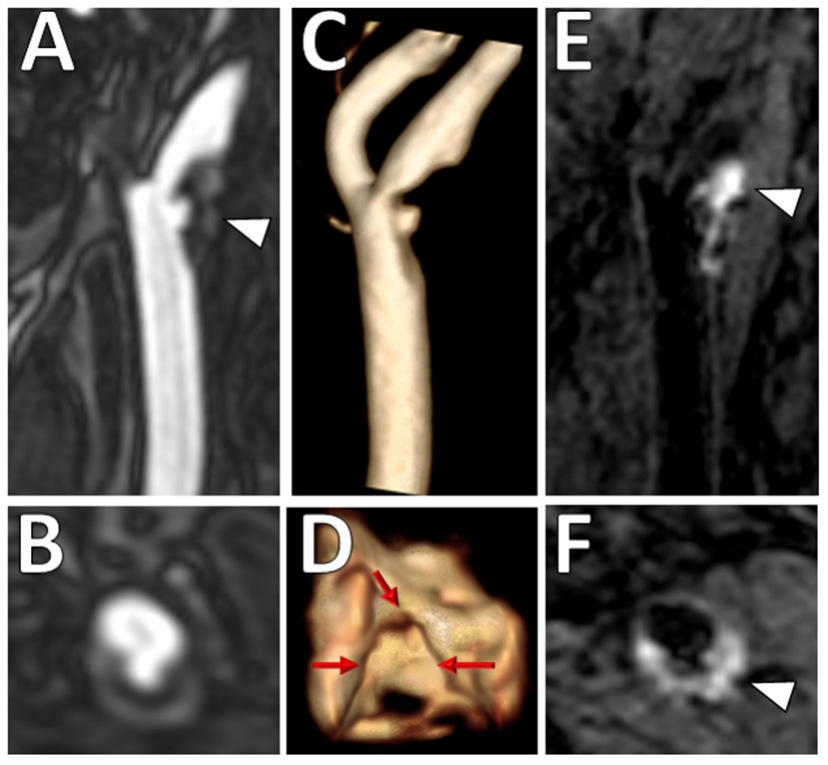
Ulcerated plaque with intraplaque hemorrhage on VW-MRI. Male patient presented with clinical and imaging evidence of a 1–2-year history of recurrent left MCA territory infarcts. (A,B) Contrast-enhanced MRA neck confirmed a left cervical carotid bifurcation stenosis with an ulcerated plaque (A, arrowhead). (C,D) 3D surface volume rendering of the left carotid plaque depicts the ulcerated surface morphology with (D) axial image through the ulceration showing marked surface irregularity (red arrows). (E,F) Carotid VW-MRI showed intraplaque hemorrhage (arrowheads), depicted as T1 hyperintense signal on a fat-suppressed T1W MPRAGE image. Based on the clinical and imaging findings, the patient underwent a left carotid endarterectomy.
